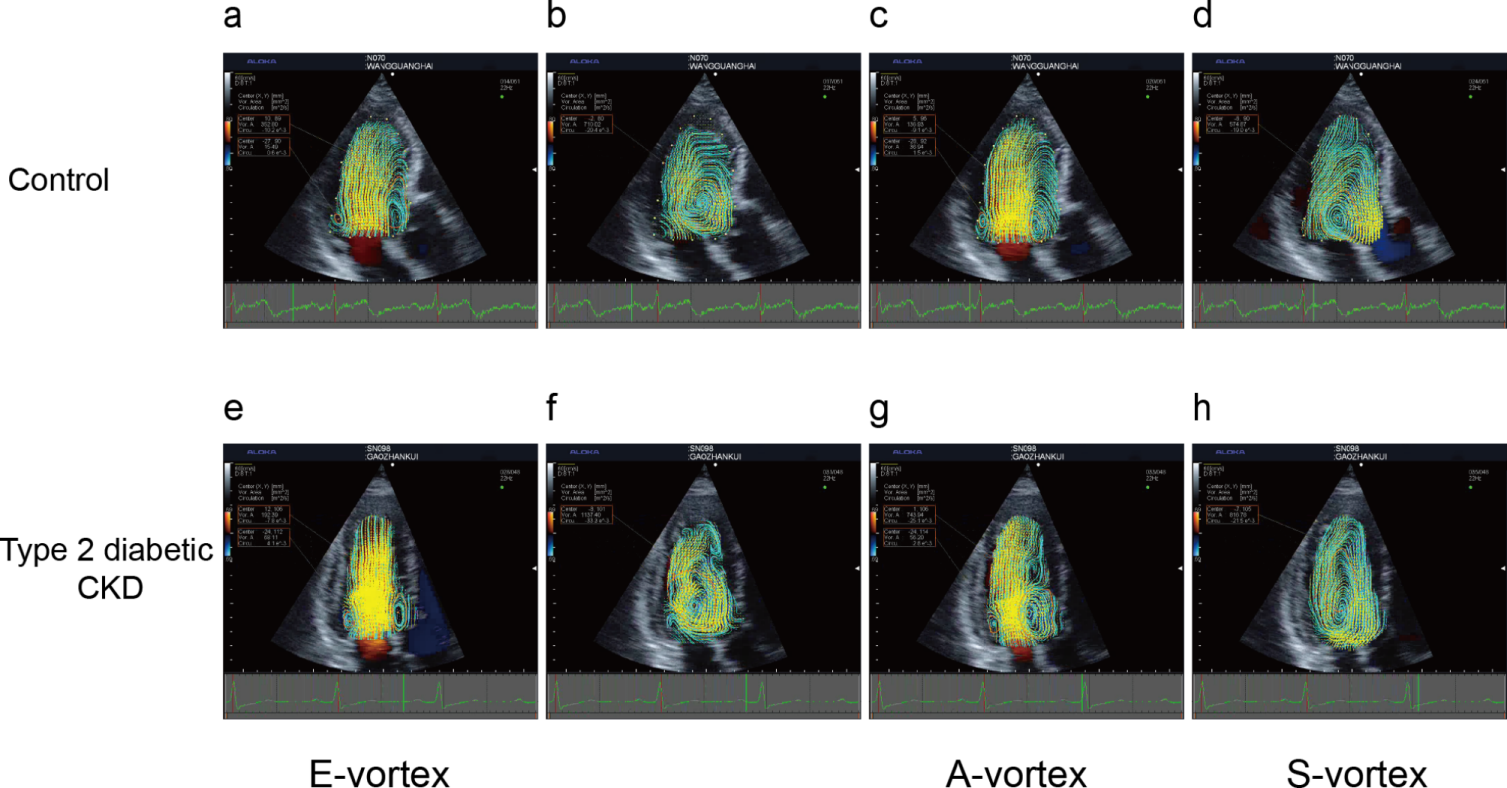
Representative LV vortex images in apical three-chamber view. Representative images of vortex during early diastole (a), vortex at the completion of passive ventricular filling (b), vortex during atrial contraction (c) and vortex during systole (d) collected from a control participant. Representative images of vortex during early diastole (e), vortex at the completion of passive ventricular filling (f), vortex during atrial contraction (g) and vortex during systole (h) collected from a type 2 diabetic patient with stages 2-3a
